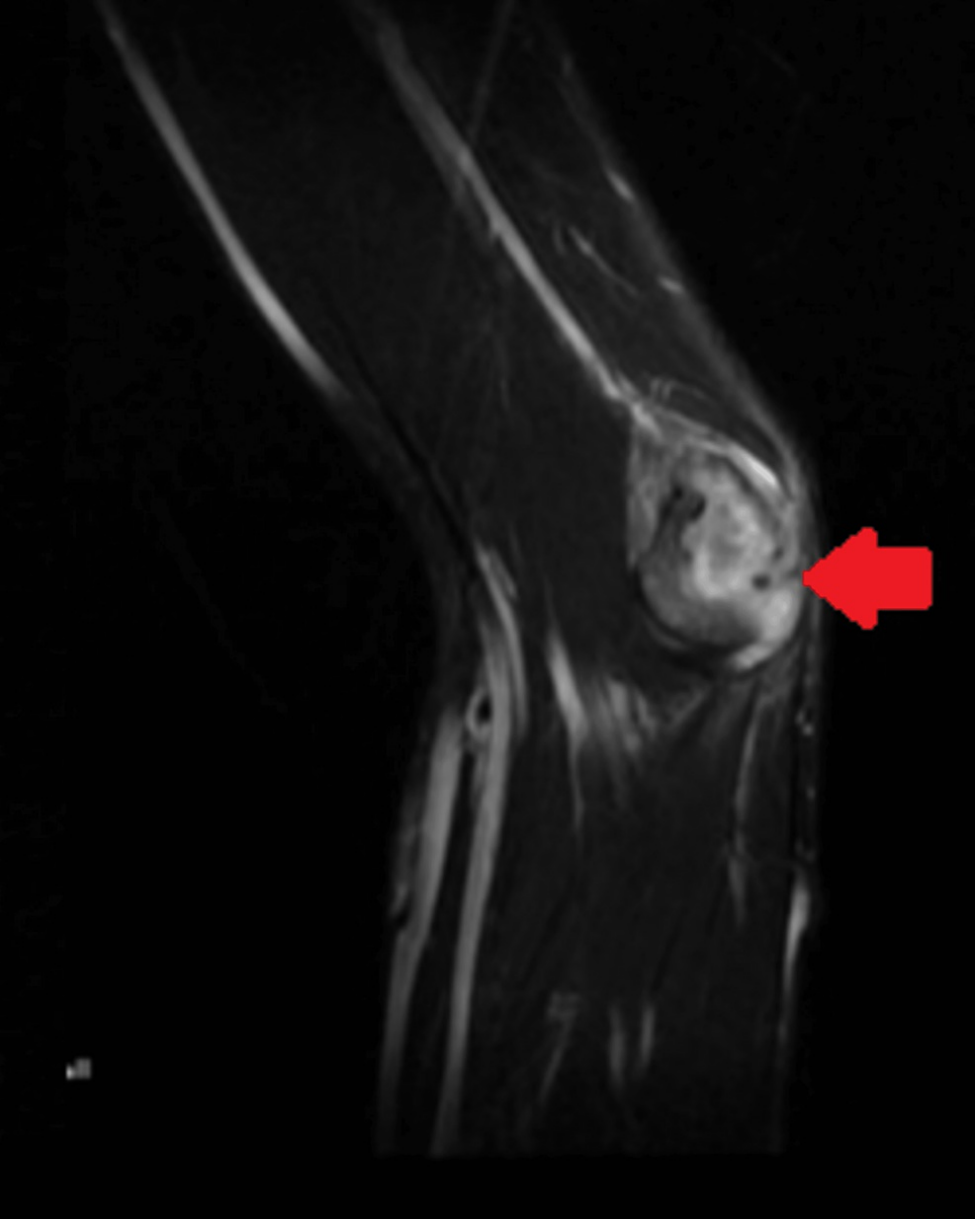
MRI of the left elbow joint Arrow showing a T1-weighted image with involvement of the left humerus MRI: magnetic resonance imaging
